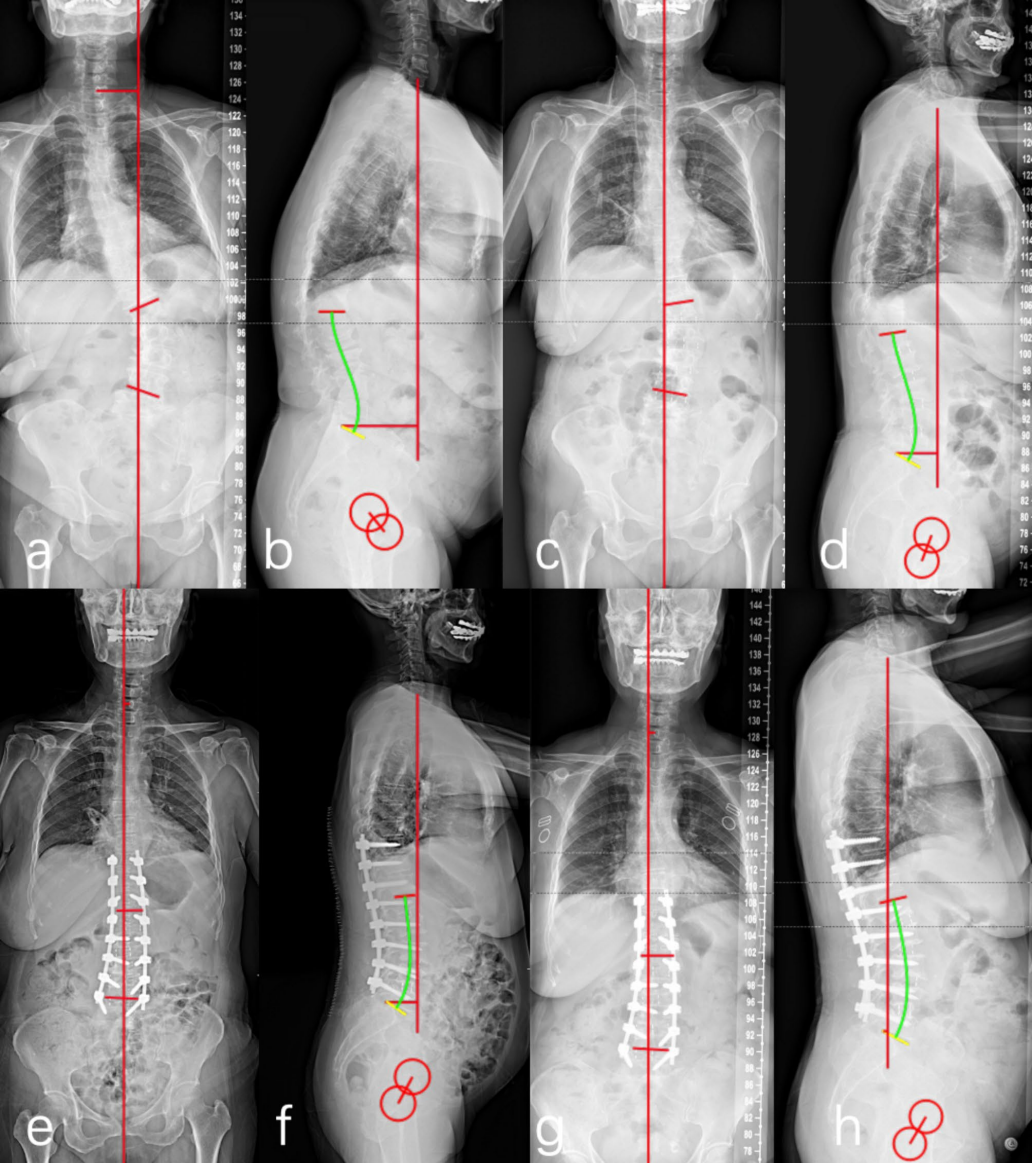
a,b Preoperative full-length anteroposterior and lateral radiographs of a 68-year-old female showing severe lumbar scoliosis and sagittal imbalance. c,d Coronal and sagittal deformities were partially corrected after the first-stage LLIF from L1/2 to L4/5. e,f Coronal and sagittal deformities were further corrected after posterior instrumented fusion from T10-L5. g,h The global and regional balance was maintained well at 16 months after surgery
